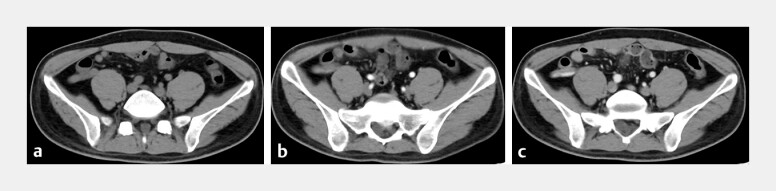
Computed tomography (CT) images of the appendiceal hemorrhage.
